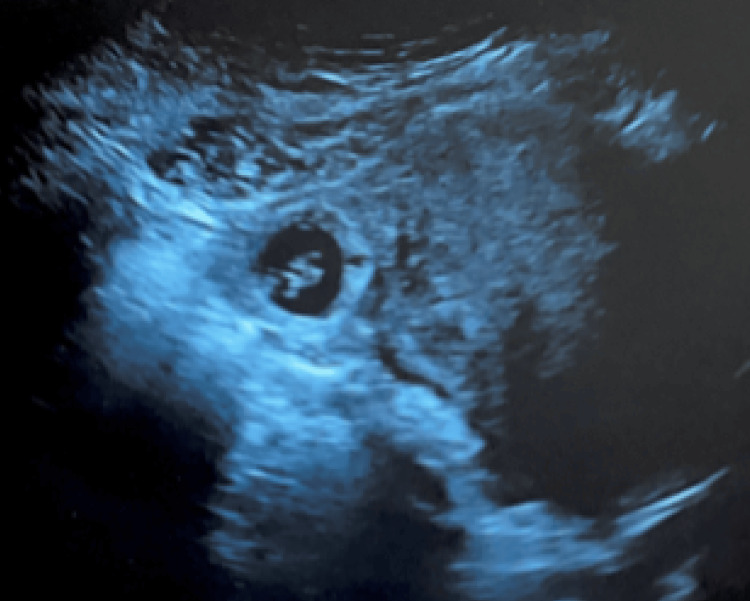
Transvaginal ultrasonography image of the uterus (transverse view) showing the ectopic pregnancy, presumably in the ampulla of the fallopian tube.
